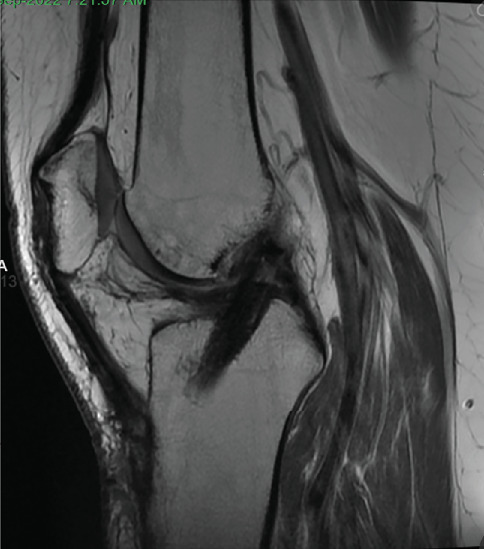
MRI from 09/2022 demonstrating intact ACL reconstruction.
